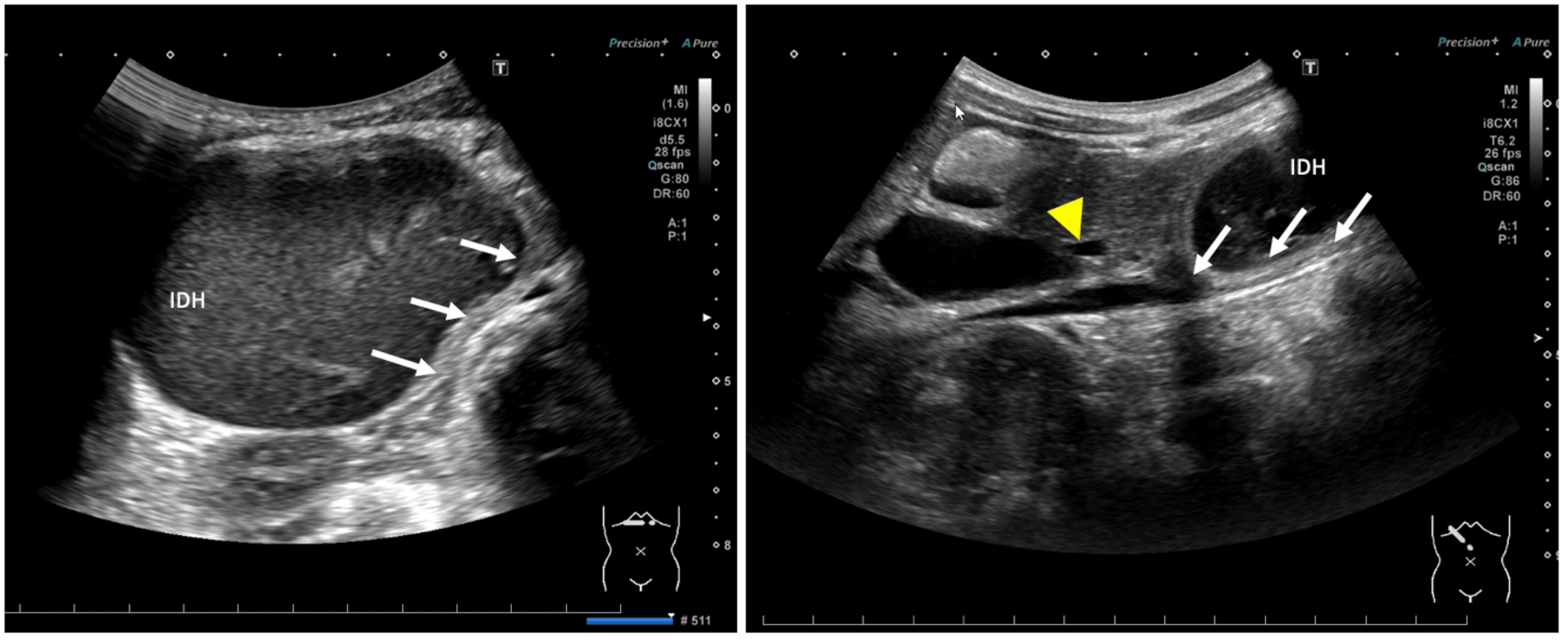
Abdominal ultrasonography. A hypoechoic mass (intramural duodenal hematoma) measuring 7.1 cm × 4.5 cm was compressing both the duodenum (arrow) and the common bile duct (arrowhead).
